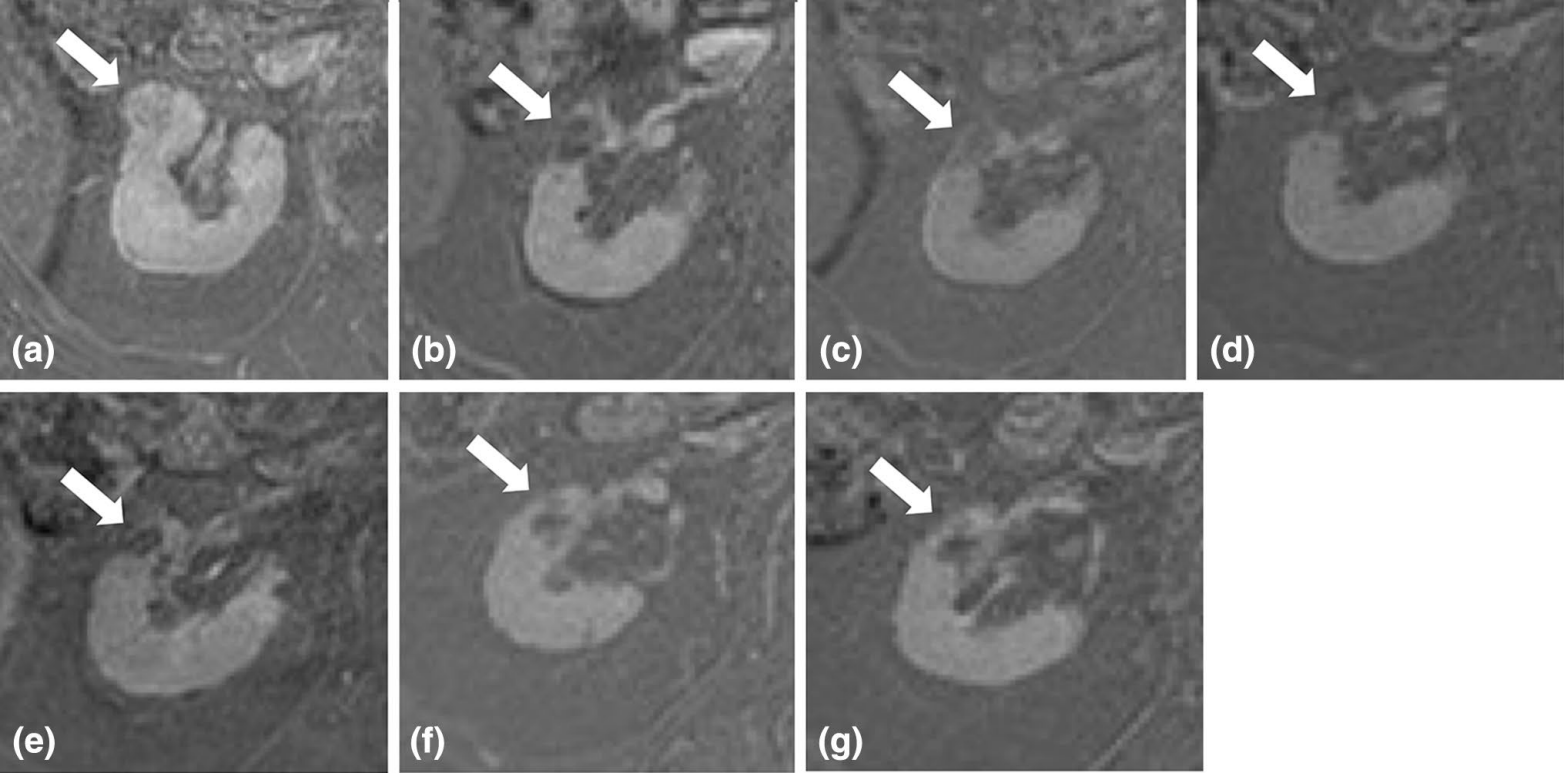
Normal CE MR imaging appearance following cryoablation of a RCC. a Pre-ablation fat-suppressed T1-weighted CE MRI shows a 2.5 cm enhancing mass in the mid portion of the right kidney, located anteriorly (arrow). b–g Follow-up CE MRI 6 months, 1 year, 2 years, 3 years, 4 years and 5 years after cryoablation show lack of enhancement within the ablation zone together with progressive shrinkage of this area (arrows)
